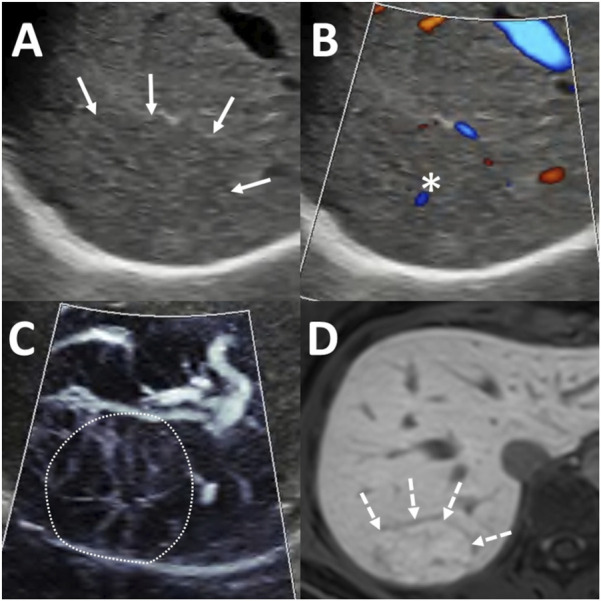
Diagnosis of focal nodular hyperplasia (FNH) using ultrasound (US) and magnetic resonance imaging (MRI). A 65 mm lesion was located beneath the diaphragm in segment 7 (A) Greyscale US showed an isoechoic lesion with indistinct margins (arrows). (B) Color-coded power Doppler imaging (PDI) highlighted the flow signal in a central artery (asterisk). (C) Microvascular imaging (MVFI) revealed a spoke-wheel vascular pattern within the lesion (outlined by the dotted line). (D) On hepatobiliary phase MRI, the lesion appeared hyperintense (dashed arrows) relative to the surrounding liver parenchyma, with a hypoenhancing central scar also visible.
